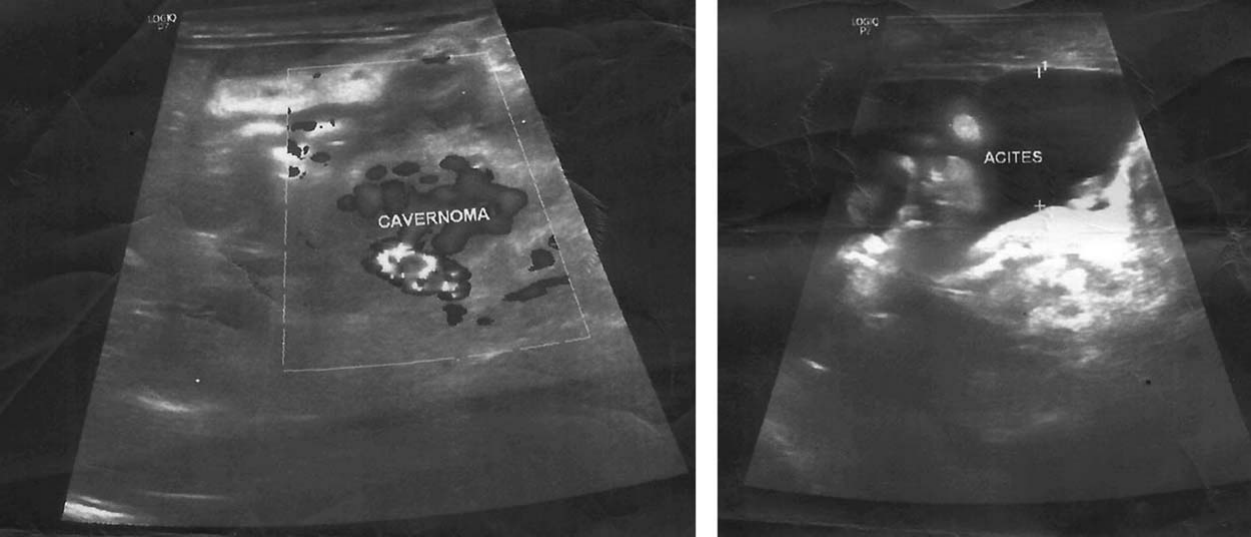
Doppler ultrasound shows impaired blood flow in the portal vein and varices in both the gastric antrum and splenic hilum.
